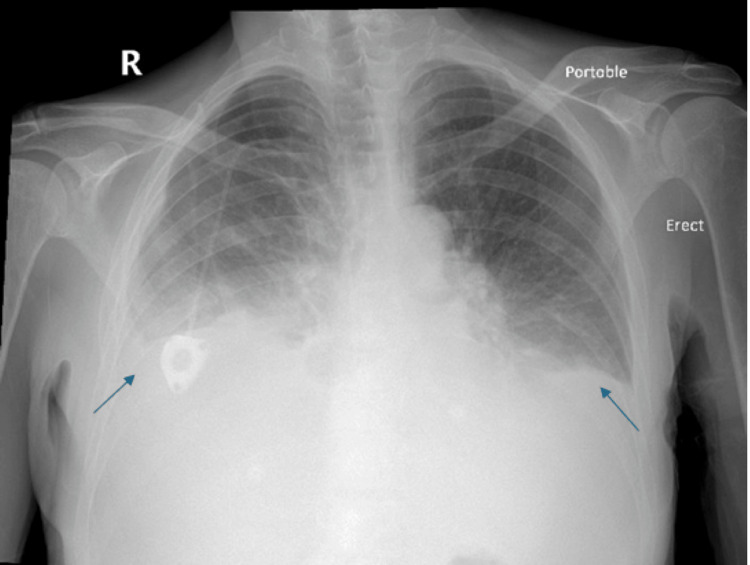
Follow-up chest X-ray one month after the initial presentation demonstrated recurrent bilateral pleural effusions (arrows).
